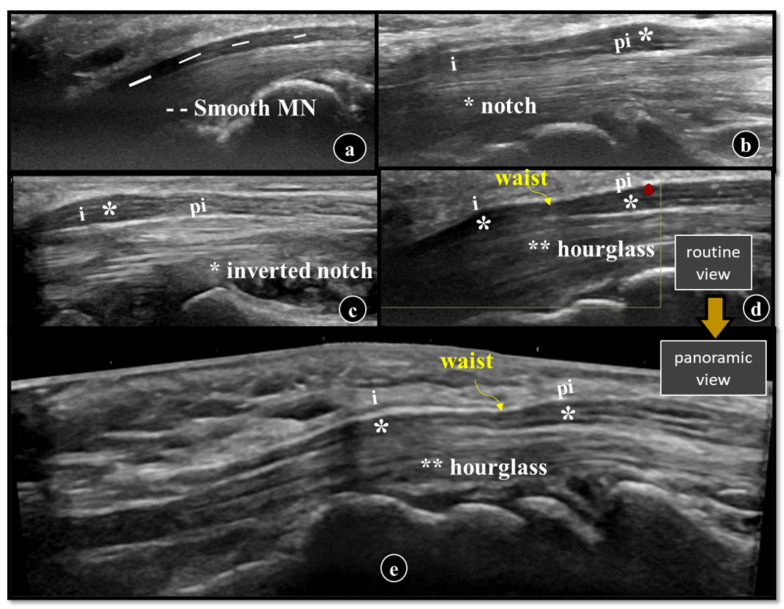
Sonographic images in longitudinal plane showing sites of swelling (*) of nerve with their corresponding signs (a–e). Normal smooth MN with no lumps and bumps (a). Nerve swelling in ‘pi’ giving notch sign (b). Nerve swelling (*) in ‘i’ or any site within inlet giving inverted notch sign (c). Nerve swelling (*) in both ‘pi’ and ‘i’ giving hourglass sign (combining two asterisks  and waist) with positive vascularity at ‘pi’ (orange dot) (d,e). Routine longitudinal (d) and panoramic view (e) showing hourglass sign. *US = ultrasound, DWC = distal wrist crease, MN = median nerve, pi = swollen nerve proximal to inlet, I = swollen nerve at inlet, * = swollen nerve.*
